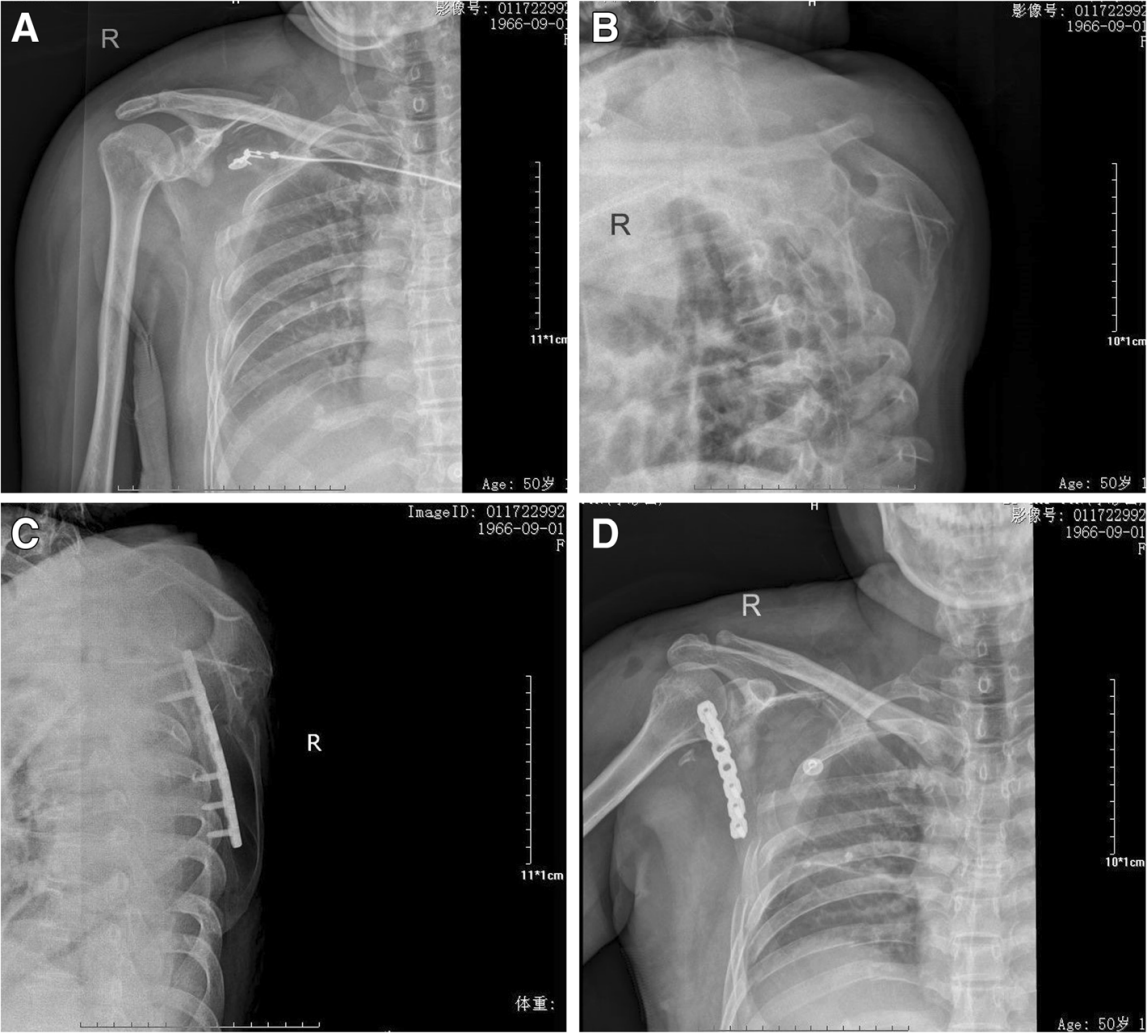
A 50-year-old female patient with fractures of the right scapula (AO 14A-3.1) accompanied by multiple fractured ribs caused by car accident. The GPA on the posteroanterior picture showed that the angle was 28.8° and facture displacement was 3.5 cm. Straight reconstruction locking plate was used for the fixation. a Posteroanterior X-ray before surgery. b Lateral X-ray before surgery. c Posteroanterior X-ray after surgery. d Lateral X-ray after surgery
